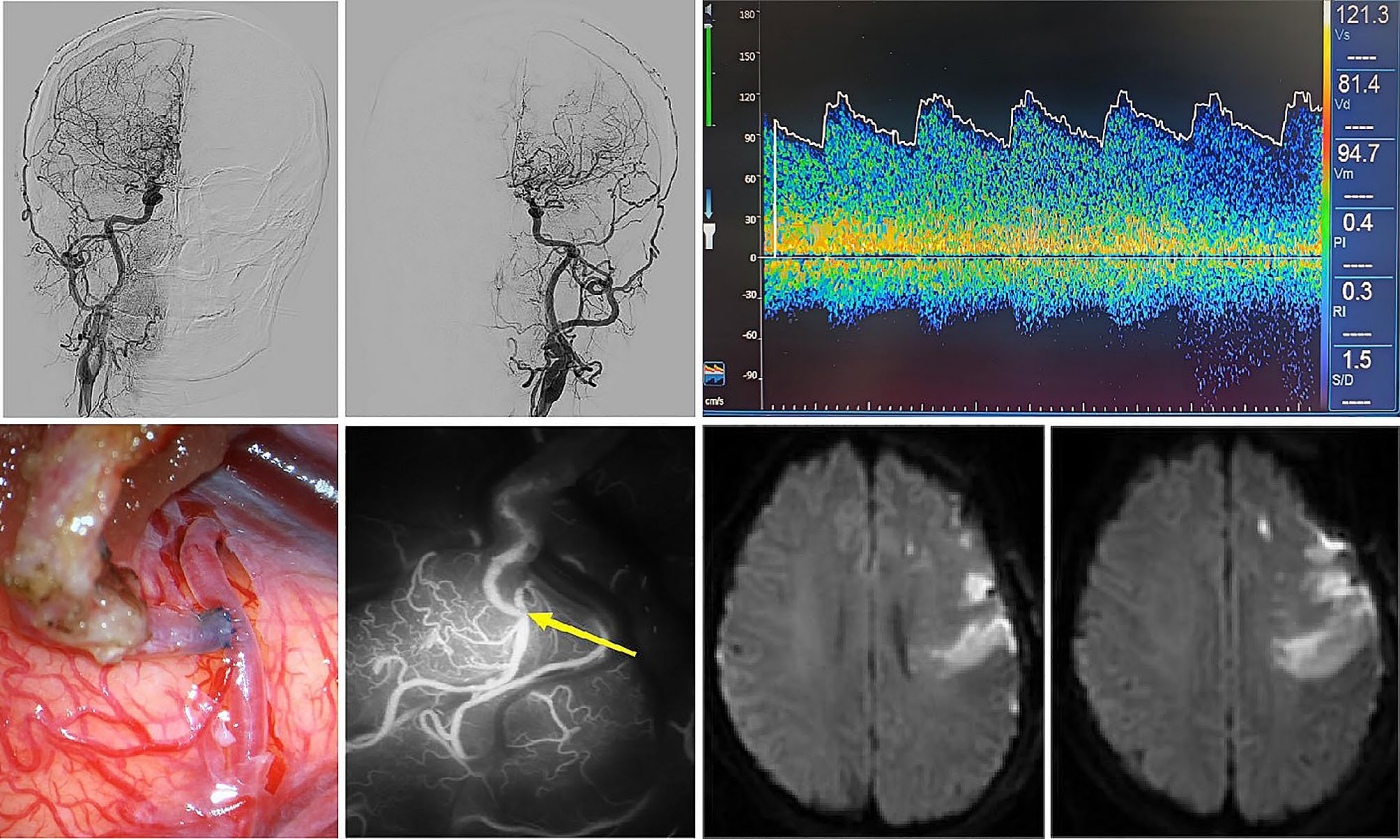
Typical case 2. This is a 42-year-old female patient, who was treated for 7 months because of intermittent speech disorder and weakness of the right limb. After admission, the patient was diagnosed as moyamoya disease by DSA examination, Suzuki stage III. TCD examination showed that the PSV, EDV and MFV of the left MCA were 121.3 cm/s, 81.4 cm/s and 94.7 cm/s. The PI was 0.422. Then the patient also underwent left STA-MCA vascular anastomosis combined with EDMS. During the operation, the anastomotic stoma was full and good, and ICG fluorescein angiography showed that the bridging vessel was unobstructed. However, on the first day after the operation, motor aphasia and grade II right limb muscle strength were observed. Immediate examination of MRI showed that the DWI sequence found the new acute cerebral infarction appeared in the frontal lobe of the operative side. The patient’s condition was gradually stable after rehydration, an appropriate pressure increase, the addition of aspirin enteric-coated tablets, and the administration of edaravone and other secondary preventive drugs for stroke. On the fifth day after operation, the patient can simply answer; Right limb muscle strength grade IV; The right hand can make a fist and move against the palm, but the movement is slow, and the fine movements of the fingers have not recovered
